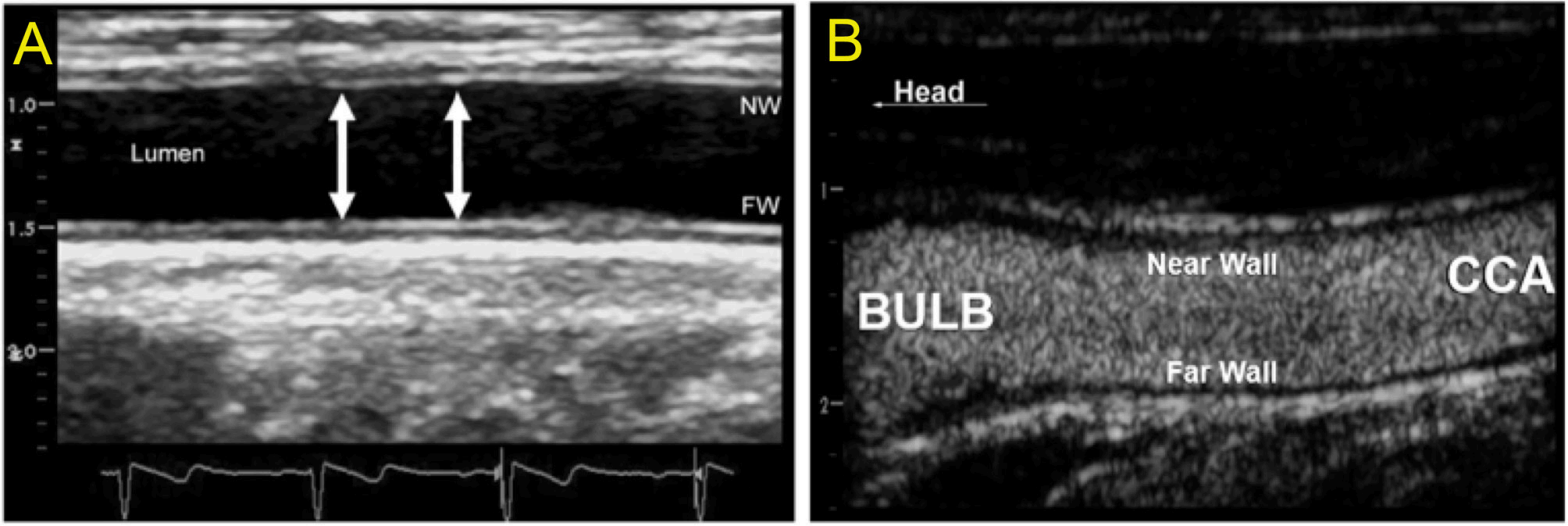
(A) Example of ultrasound-acquired images of the common carotid with B-mode non-contrast-enhanced ultrasonography and visualization of intima-media thickness in the near wall (NW) and far wall (FW); (B) example of near wall and far wall visualization using contrast‐enhanced ultrasound imaging. Reproduced from Shah BN, Chahal NS, Kooner JS & Senior R; Contrast‐enhanced ultrasonography vs B‐mode ultrasound for visualization of intima‐media thickness and detection of plaques in human carotid arteries; *Echocardiography* 2017, volume 34, pages 723–730 (33). Copyright 2017 John Wiley and Sons.
